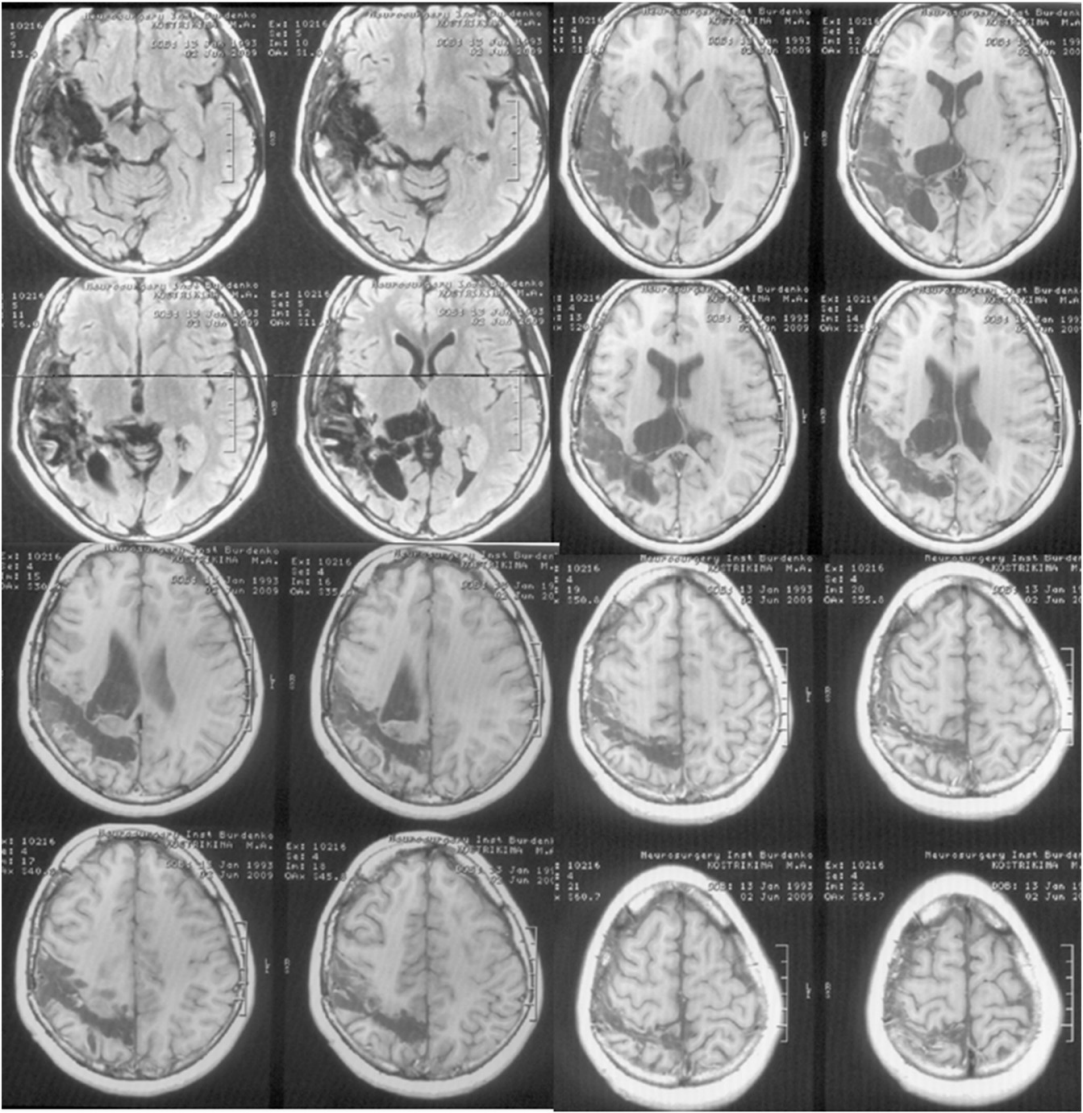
Postoperative MRI. Volume of surgical intervention (described in the text of the article).
